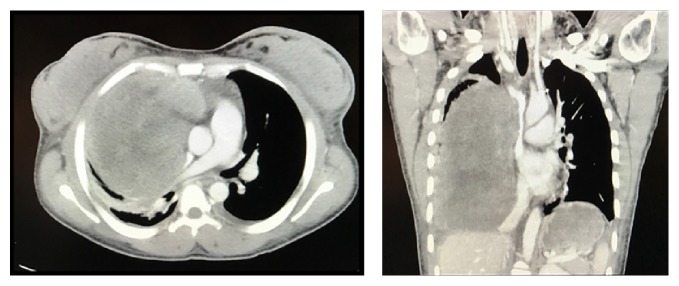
Chest CT showing the superior vena cava obstruction.
